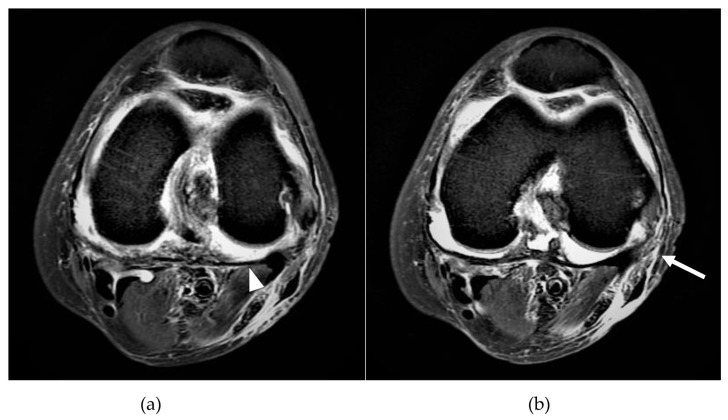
A 48-year-old male on regular hemodialysis presented to the emergency department with fever, left knee pain and swelling. The patient underwent T2-weighted fat-suppressed magnetic resonance imaging: (a) The homogenous signal in the left knee articular space was compatible with synovial effusion (arrowhead). (b) The synovial thickening and proliferation were consistent with inflammation (arrow).
